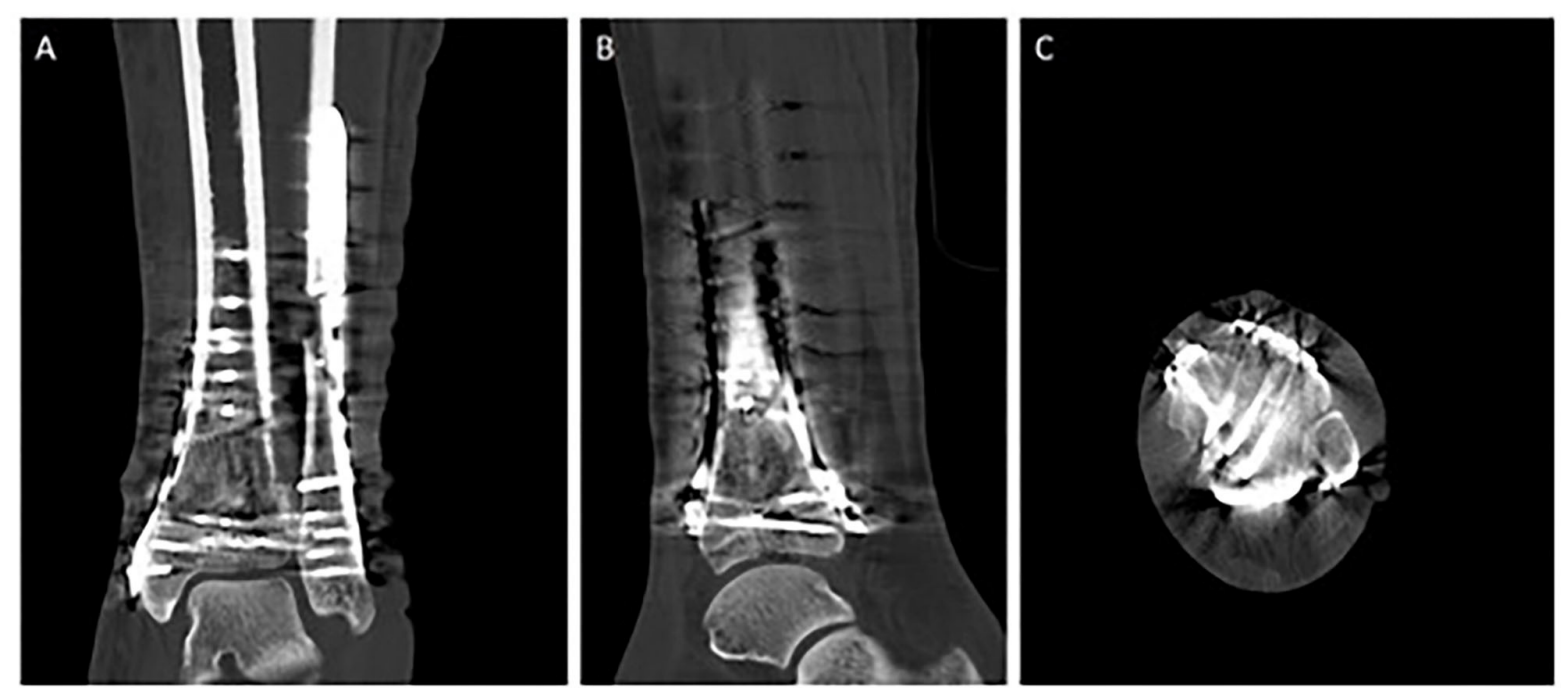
Triple plate ORIF of a tibial pilon fracture AO type 43-C3. Postoperative CT scans [(A) coronal plane, (B) sagittal plane, (C) axial plane] of a 49-year-old female patient following operative treatment of an AO type 43-C3 tibial pilon fracture, resulting from a hiking accident. As a first step, fracture reconstruction was started with a posterolateral approach to address the dorsal tibial component with a buttress plate. The comminuted fibula was restored in length and rotation afterwards. As a second step, the extensile approach was used to address the anterior and the anteromedial component with two angular stable plates.
